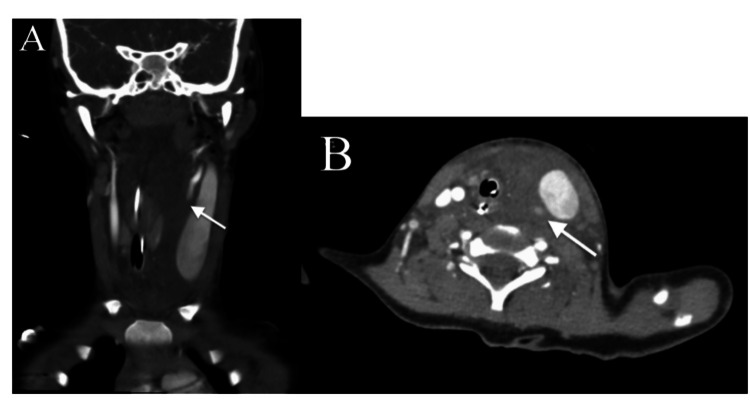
CT angiogram neck from hospital day 0 demonstrating loss of opacification of the left carotid artery with surrounding hematoma and resultant left jugular venous distension and tracheal deviation to the right in coronal (A) and axial (B) planes.
